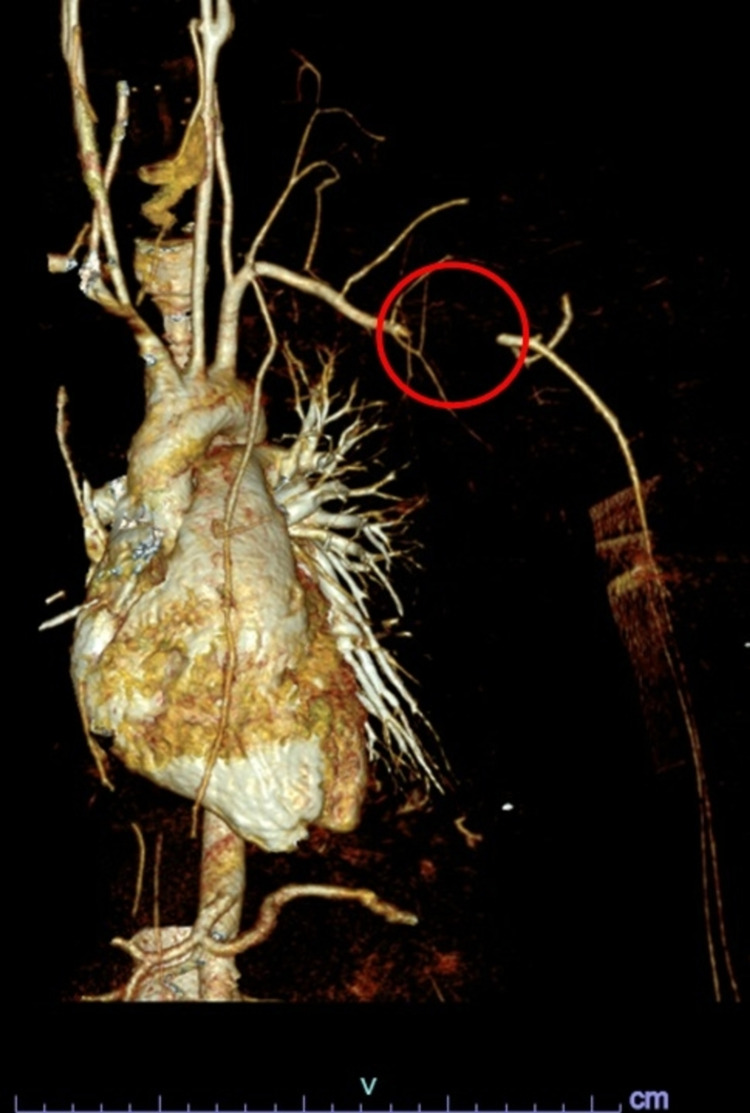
Three-dimensional CT angiography after the initial trauma showing abrupt loss of opacification of the left subclavian artery for 2.9 cm in length and then distal reconstitution of the left subclavian artery (circled).
